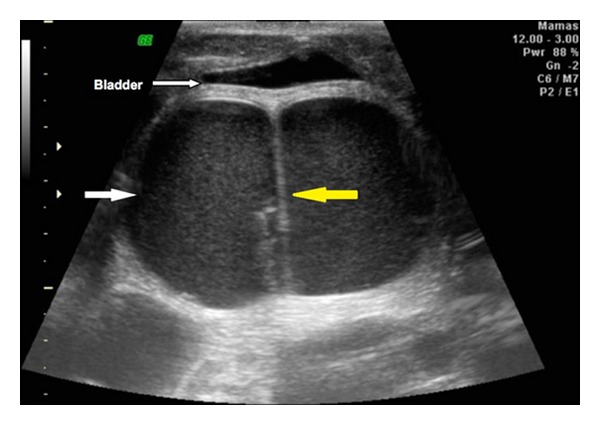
Ultrasound scan in axial plane at the level ofthe fetal pelvic region, shows the bladder brought forward by the cystic mass of dense content (white arrow), at the midsagittal septum (yellow arrow).
